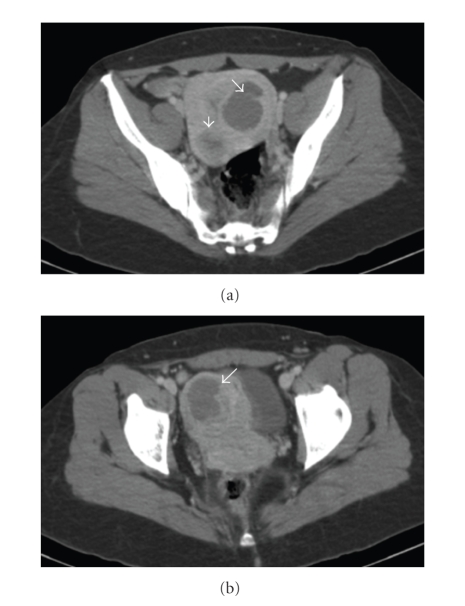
CT-scan of the abdomen, performed for GTN staging after two cycles of interval systemic methotrexate for hydatidiform mole. White arrows indicate the presence of three fibroids, resulting in important distortion of the uterine cavity. At this stage, no residual molar tissue was observed radiologically.
